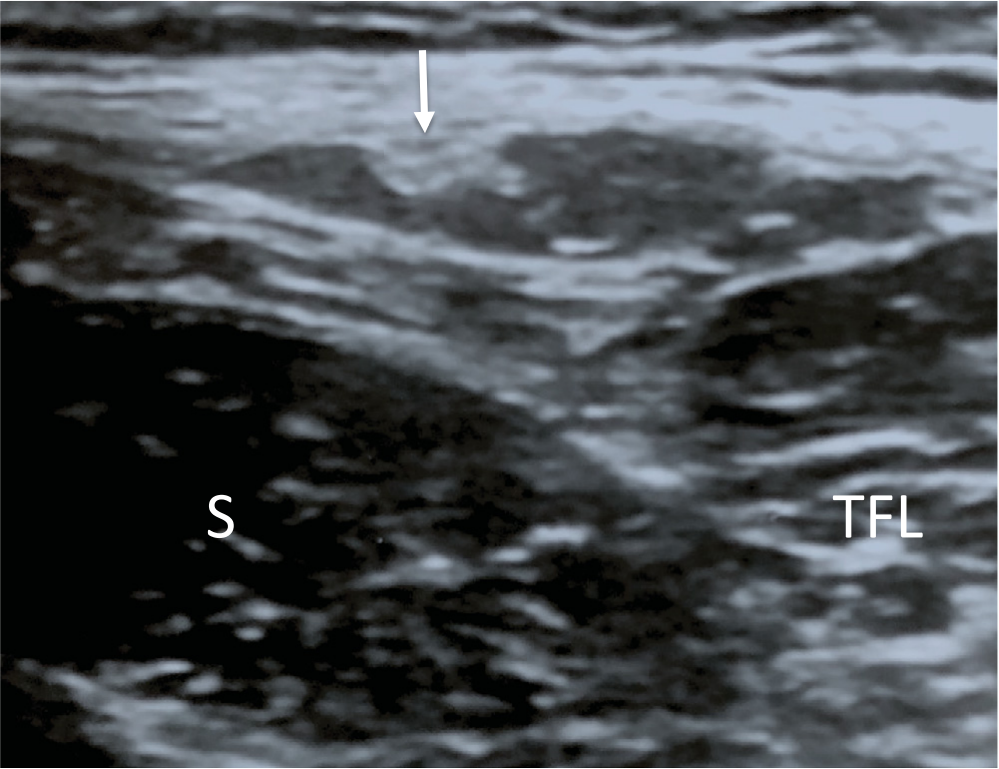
Transverse ultrasound image of the Non-Fan type within the intermuscular space between the sartoirus and the tensor fascia latae. S: Sartorius, TFL: tensor fascia latae muscle, Arrow: nerve branch.
